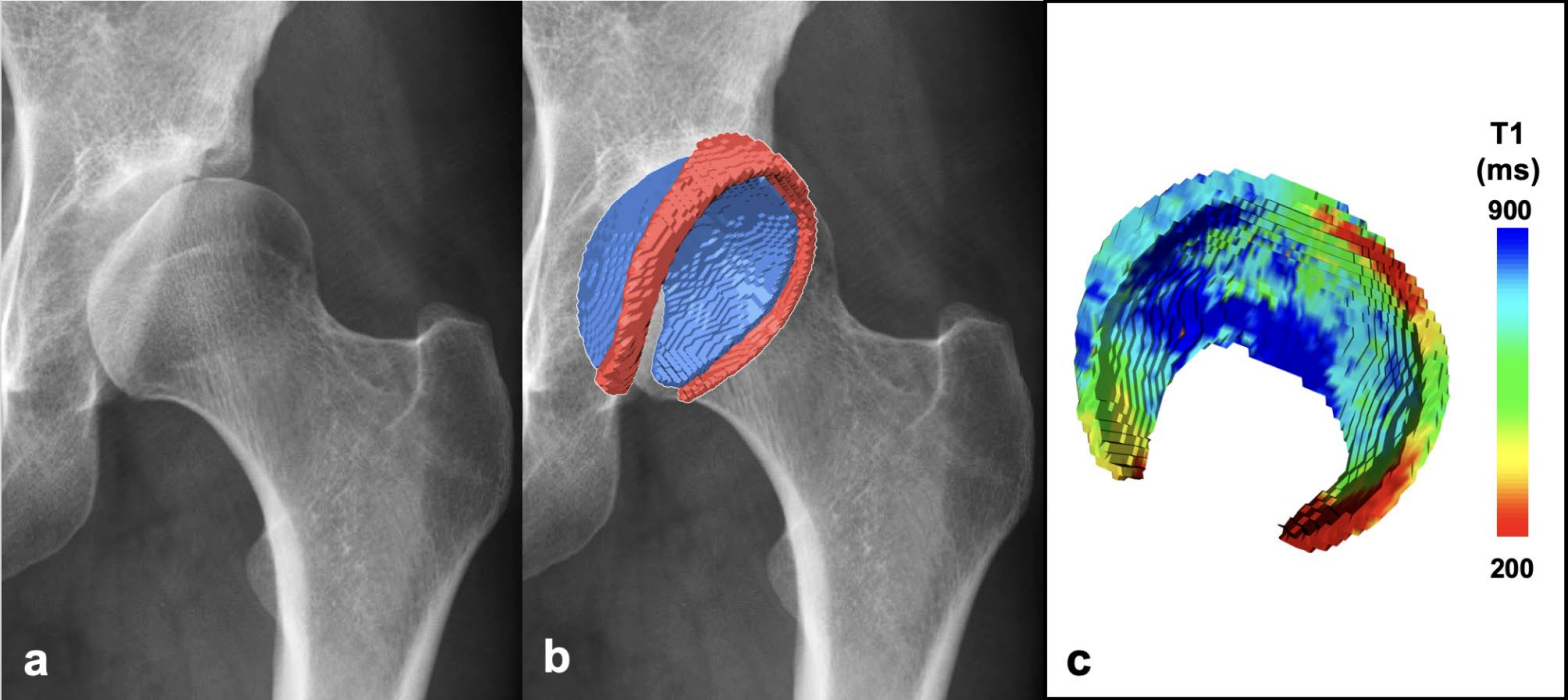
Patient example. (a) Antero-posterior radiograph of a 29 year old patient with hip dysplasia. (b) 3D morphologic models of hip cartilage and labrum demonstrate a large labrum volume with 1998 mm^3^ (cartilage volume = 5941 mm^3^, relative labrum volume = 34%), indicating labrum hypertrophy to compensate for deficient acetabular coverage. (c) 3D cartilage model with color coded T1 mapping demonstrate the typical dysplastic wear pattern of peripheral joint damage antero-superiorly indicated with lower T1 relaxation time (red color). 3D models were visualized using a custom-made software application for automatic segmentation and visualization^8^.
